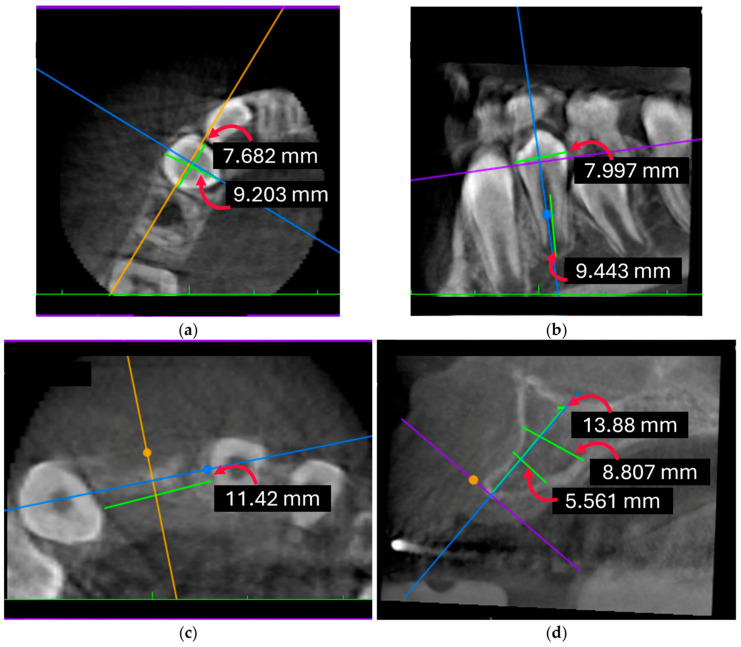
CT scans performed before surgery (December 2020): donor 44 axial section (a), donor 44 sagittal section (b), recipient site 11 axial section (c), and recipient site 11 sagittal section (d). Relevant dimensions of donor and recipient site are shown as green lines, with corresponding measurements.
